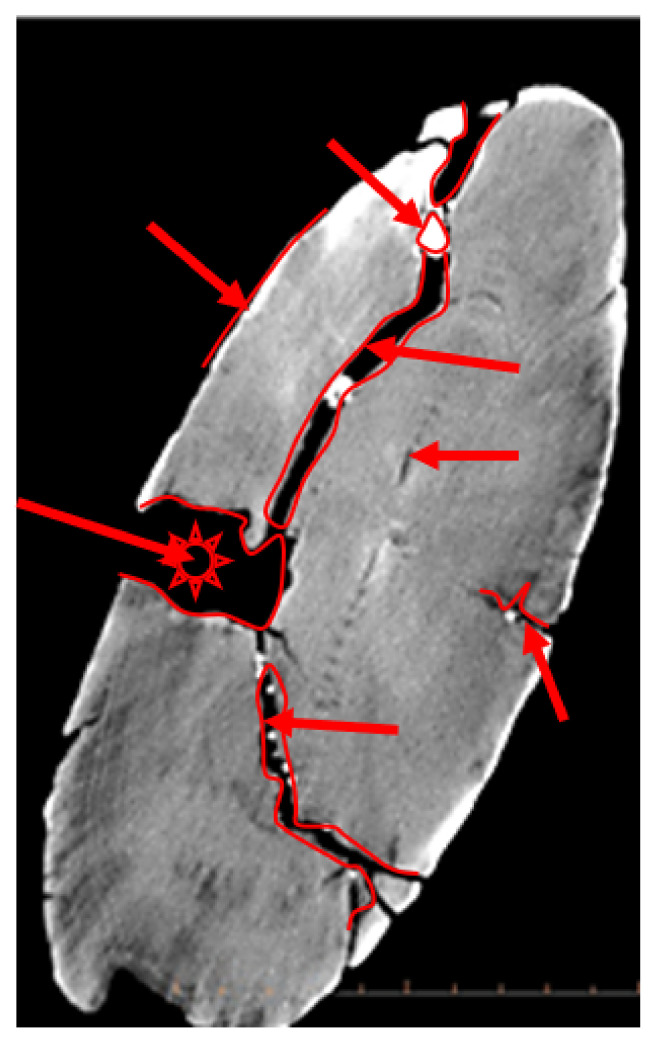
In the 2D CT image the edges of larger openings, fractures, pores, inclusions, etc., are detected first.
